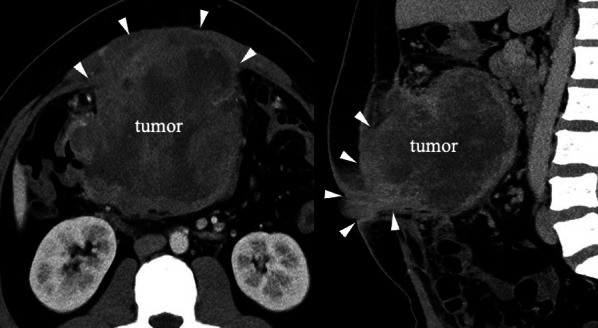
Contrast-enhanced CT scan findings at initial examination. Enhanced CT shows a large tumor with extensive direct invasion (approximately 12 cm, white arrowhead) of the anterior abdominal wall
